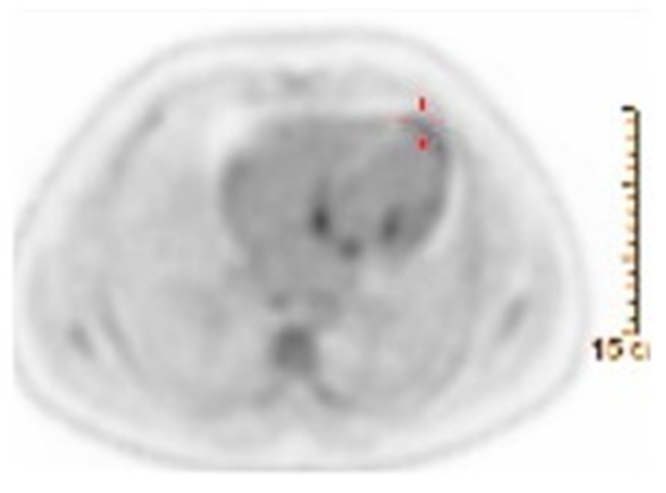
Repeat Positron Emission Tomography/Computed Tomography Demonstrating Partial Reduction of Myocardial Inflammatory Activity (Maximum Standardized Uptake Value 4.4) A new course of prednisone 80 mg/d was initiated for 3 weeks, followed by gradual tapering.
